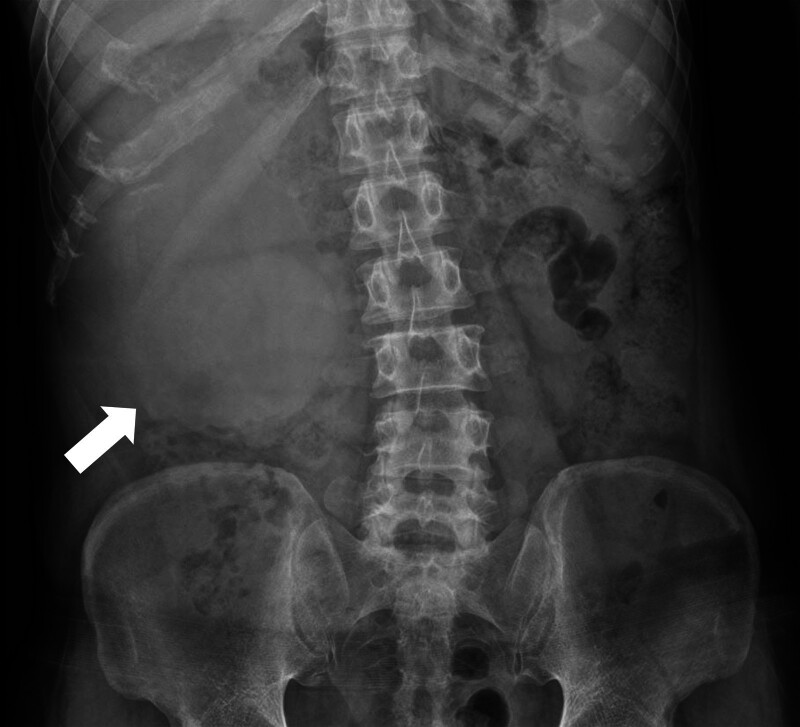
Abdominal radiography, anterior–posterior view, of this patient, showing a mass-like opacity (arrow) in the right abdomen.
